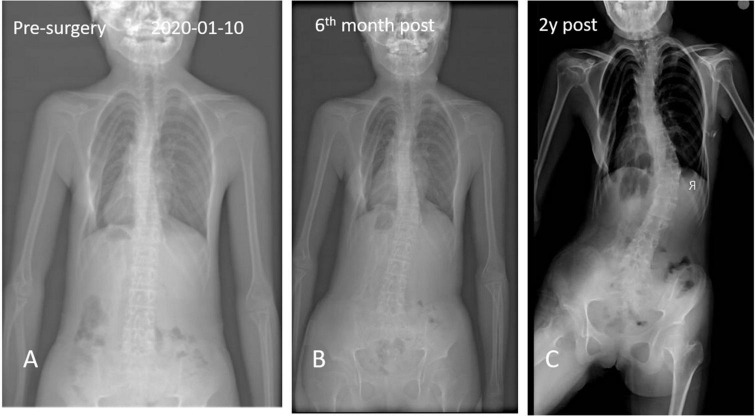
Anteroposterior and axial x-ray radiographic evaluation showed a massive radiopaque formation located predominantly in the region of back and spinal scoliosis.
